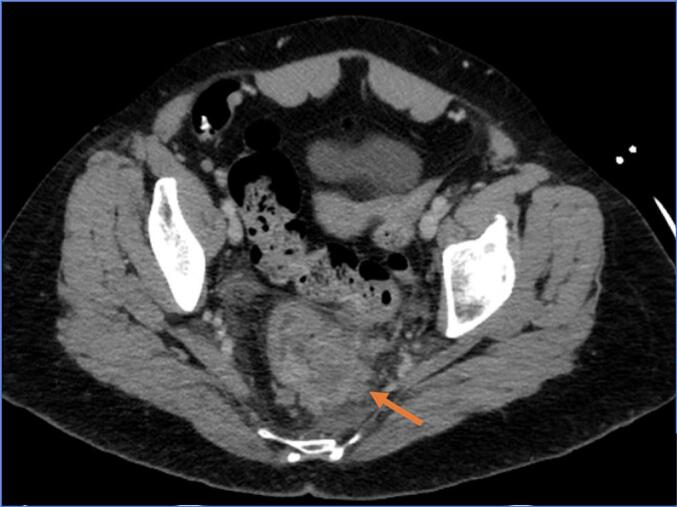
Rectal tumour on CT scan (arrow).
